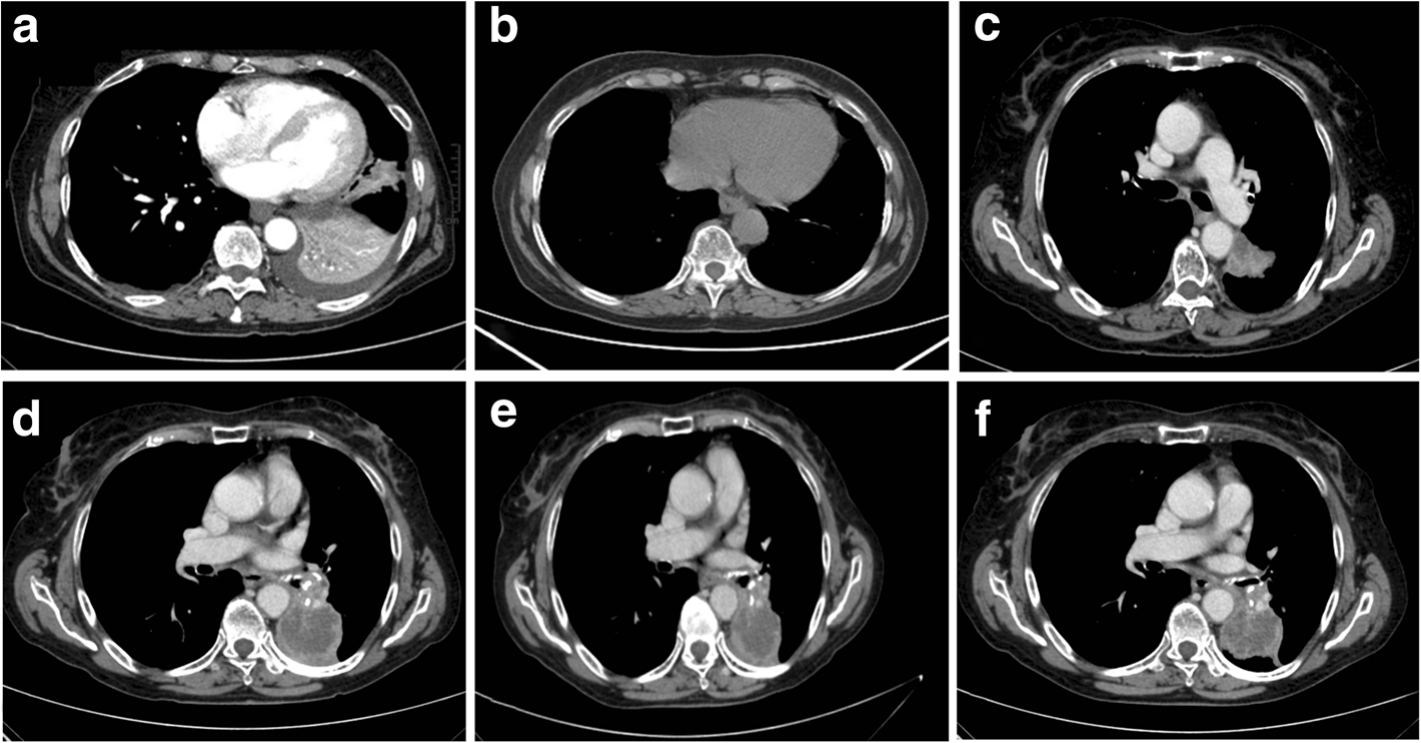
Chest CT-scan performed at diagnosis in October 2013 (a), in May 2014 following first line chemotherapy (b), in April 2015 at tumor progression following radiotherapy (c), in January 2016 before starting immunotherapy (d), in May 2016 during immunotherapy (e) and in December 2017 following 38 cycles of immunotherapy administration (f)
